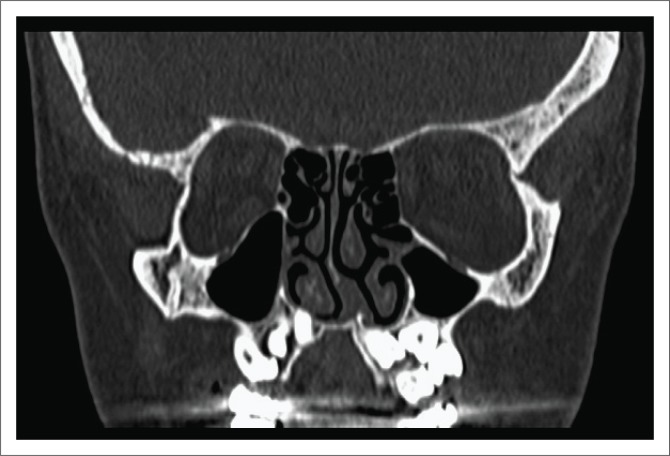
Coronal section of computed tomography-paranasal region shows supernumerary unerupted teeth, a deviated nasal septum, hypoplastic maxillary sinuses and a high-arched palate.
